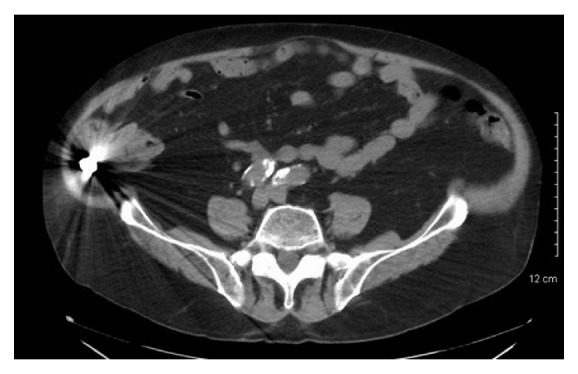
A CT abdomen and pelvis without contrast demonstrating the dental bridge persistently in the base of the cecum.
